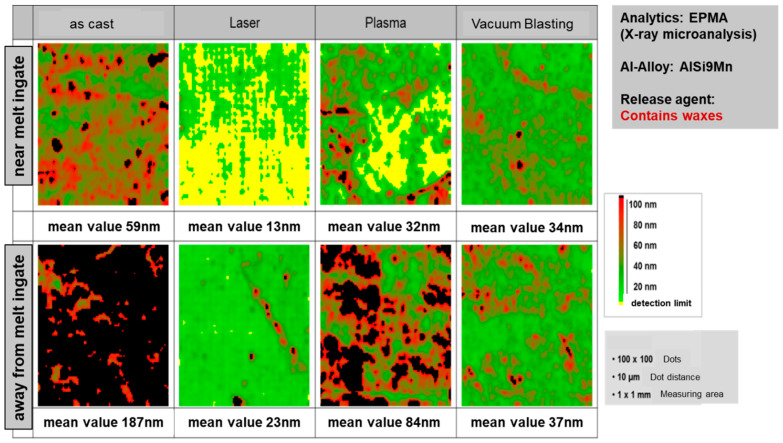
EPMA (X-ray microanalysis; carbon content) of the aluminum die-cast surface after various pre-treatments. Adapted from [164].
